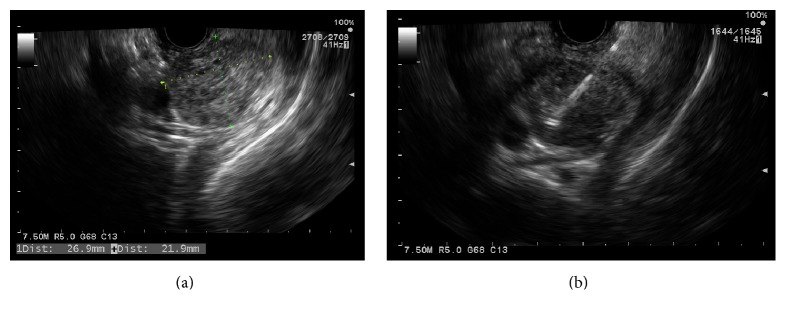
(a) EUS examination of the thyroid revealed a hypoechoic, round, and well-demarcated mass that measured 26.9 mm × 21.9 mm in the right lobe. (b) Under echoendosonographic guidance, FNA with a 25-gauge needle (Expect EUS-FNA 25 G needle; Boston Scientific, Menomonie, WI, USA) was performed successfully.
